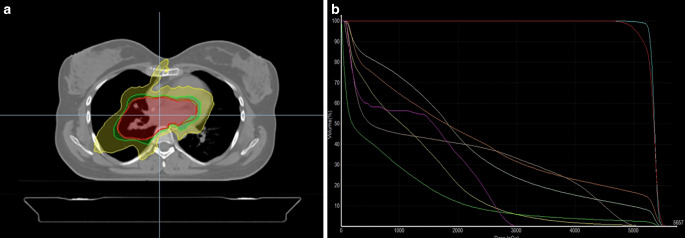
Radiation therapy plan of the presented case. a Computed tomography-based plans of intensity-modulated radiotherapy showing isodose levels in color washes. *Red*, *green*, and *yellow* colors encompass the areas covered by 95%, 80% and 50% of the prescribed dose, respectively. b Dose–volume histogram of the treatment plan. *Green*, *yellow*, *light pink*, *light green*, *magenta*, *orange*, *red*, and *turquoise*
